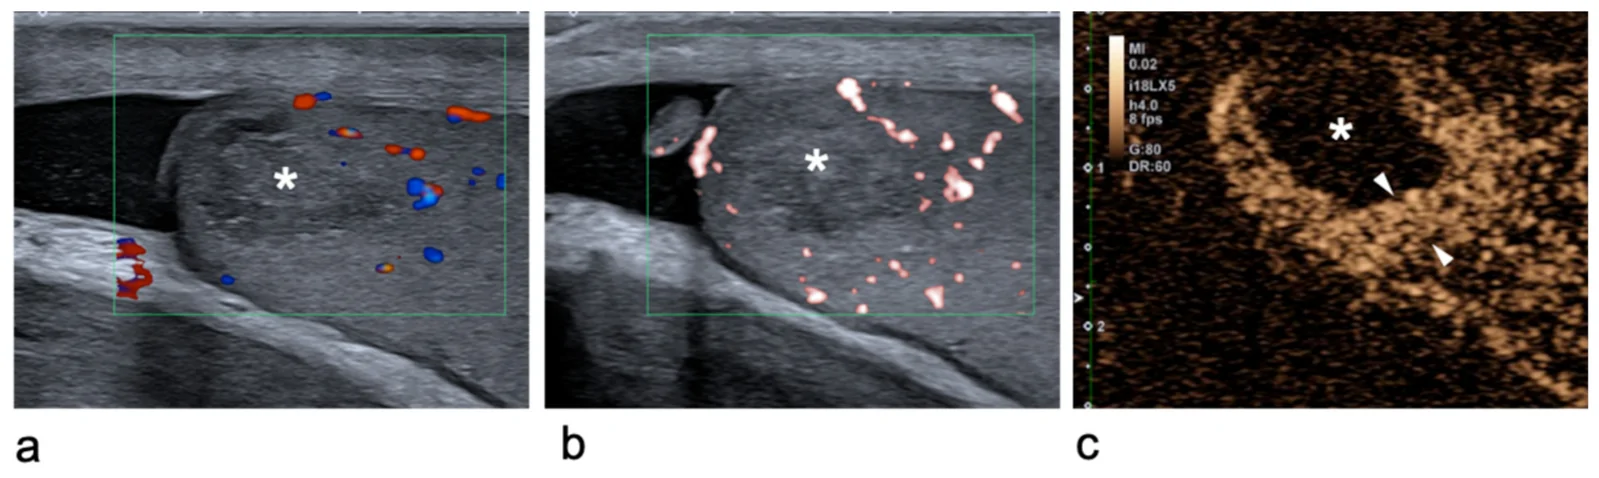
A 49-year-old man underwent ultrasonography following an episode of pain in the left testis one week earlier. He was asymptomatic at the time of examination. (a,b) Ultrasound shows a heterogeneously hypoechoic lesion (asterisk) at the upper pole of the left testis, with no vascularity on colour Doppler (a) or microvascular imaging (b). The absence of vascularity (asterisk) is confirmed on CEUS (c), which also demonstrates a rim of perilesional enhancement (arrowheads), suggestive of subacute segmental testicular infarction.
